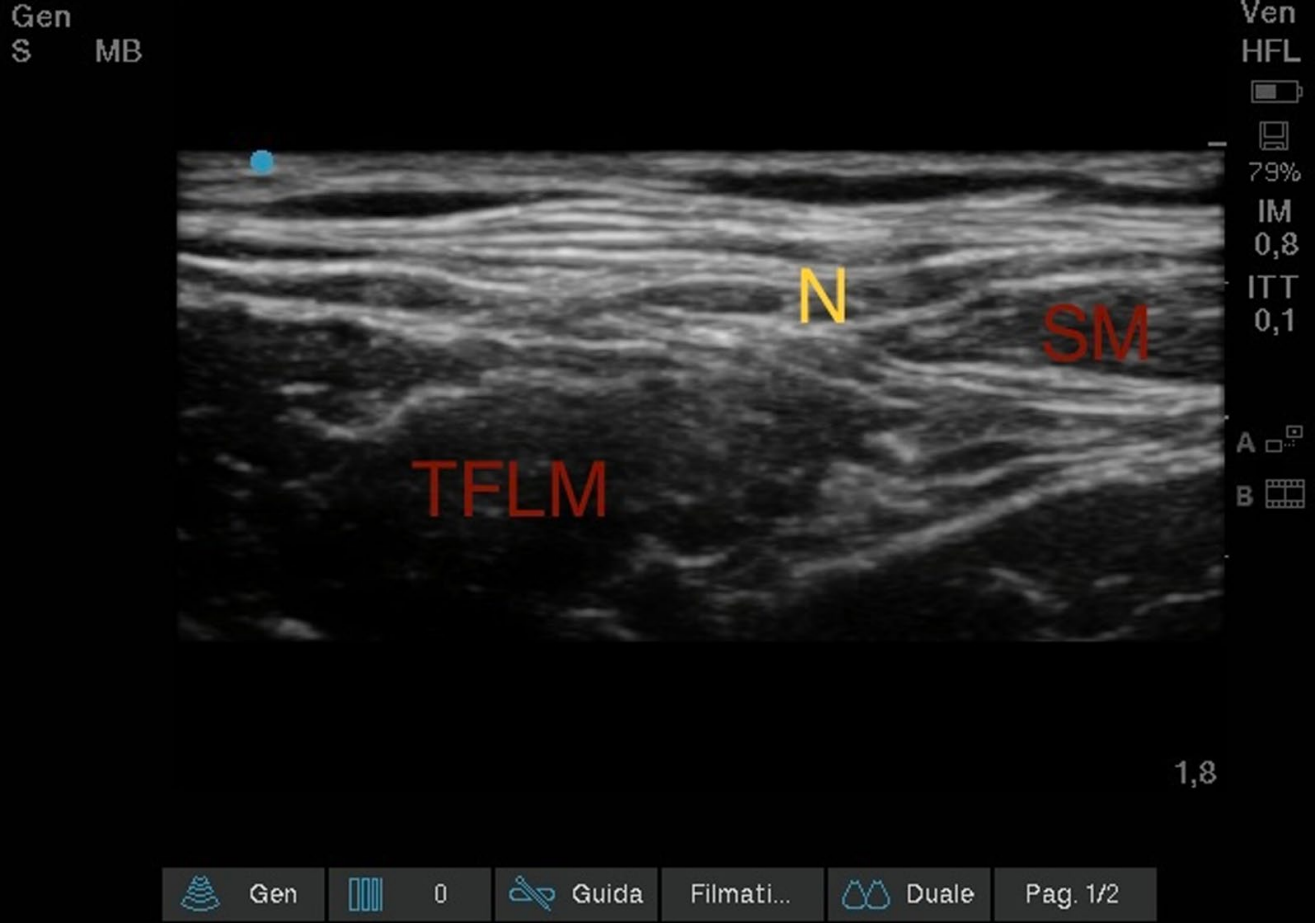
Ultrasound Lateral Femoral-Cutaneous Nerve Block. Sartorius muscle (SM); Tensor fasciae latae muscle (TFLM); Lateral Femoral-Cutaneous nerve (N)
